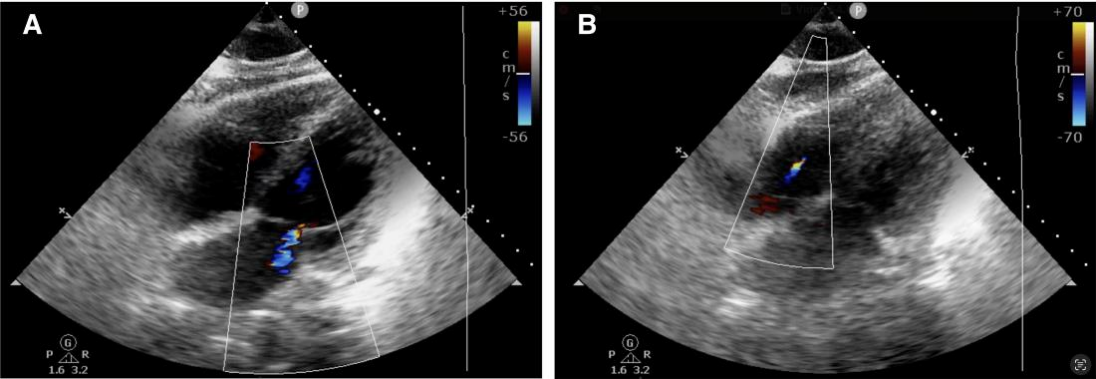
Transoesophageal echocardiography showed severe mitral regurgitation and tricuspid regurgitation nearly resolved after re-administration of sedation and analgesia (A and B)
